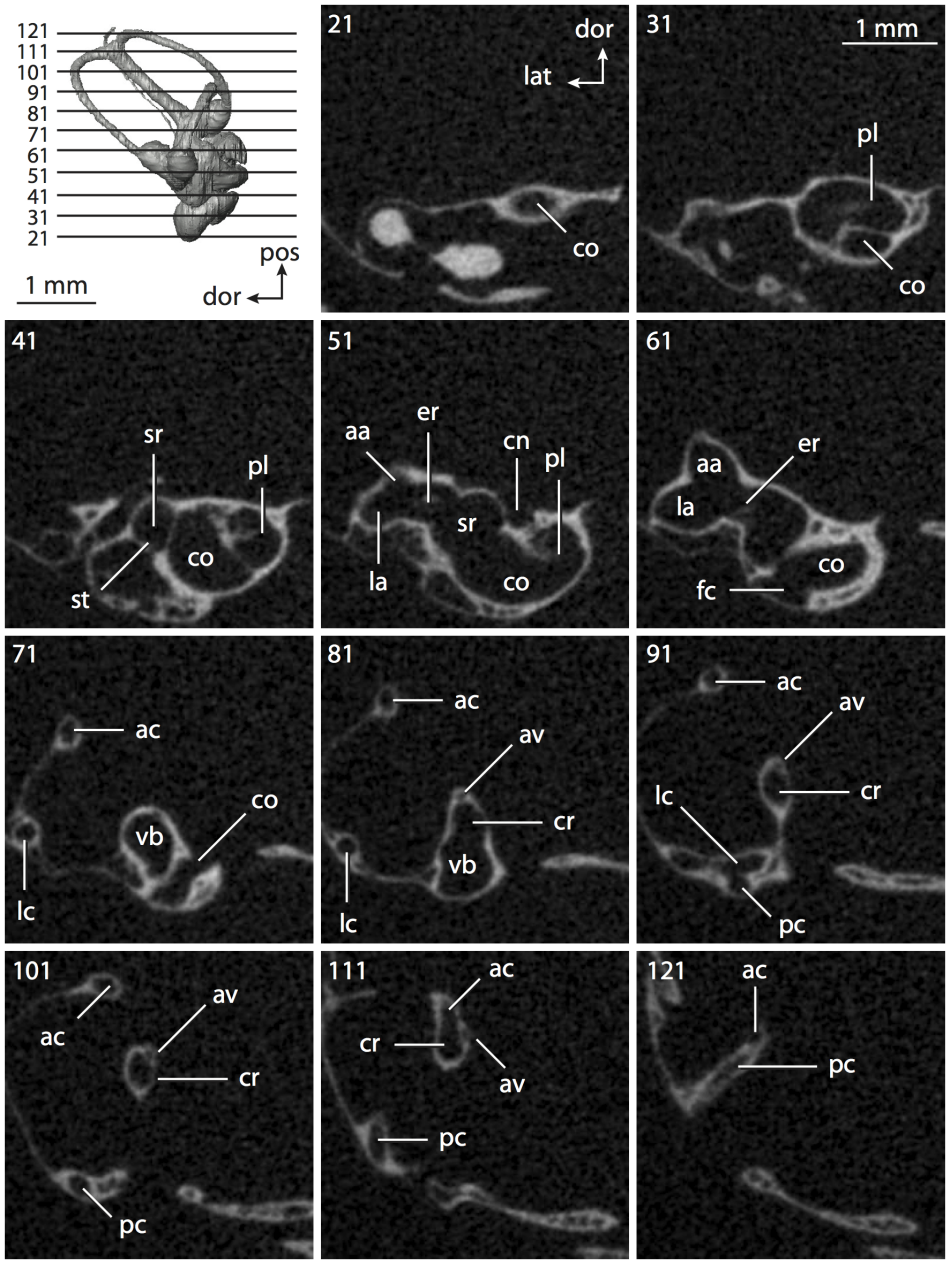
CT slices through ear region of*Sorex monticolus*. Abbreviations listed at the end of the Materials and Methods section.
